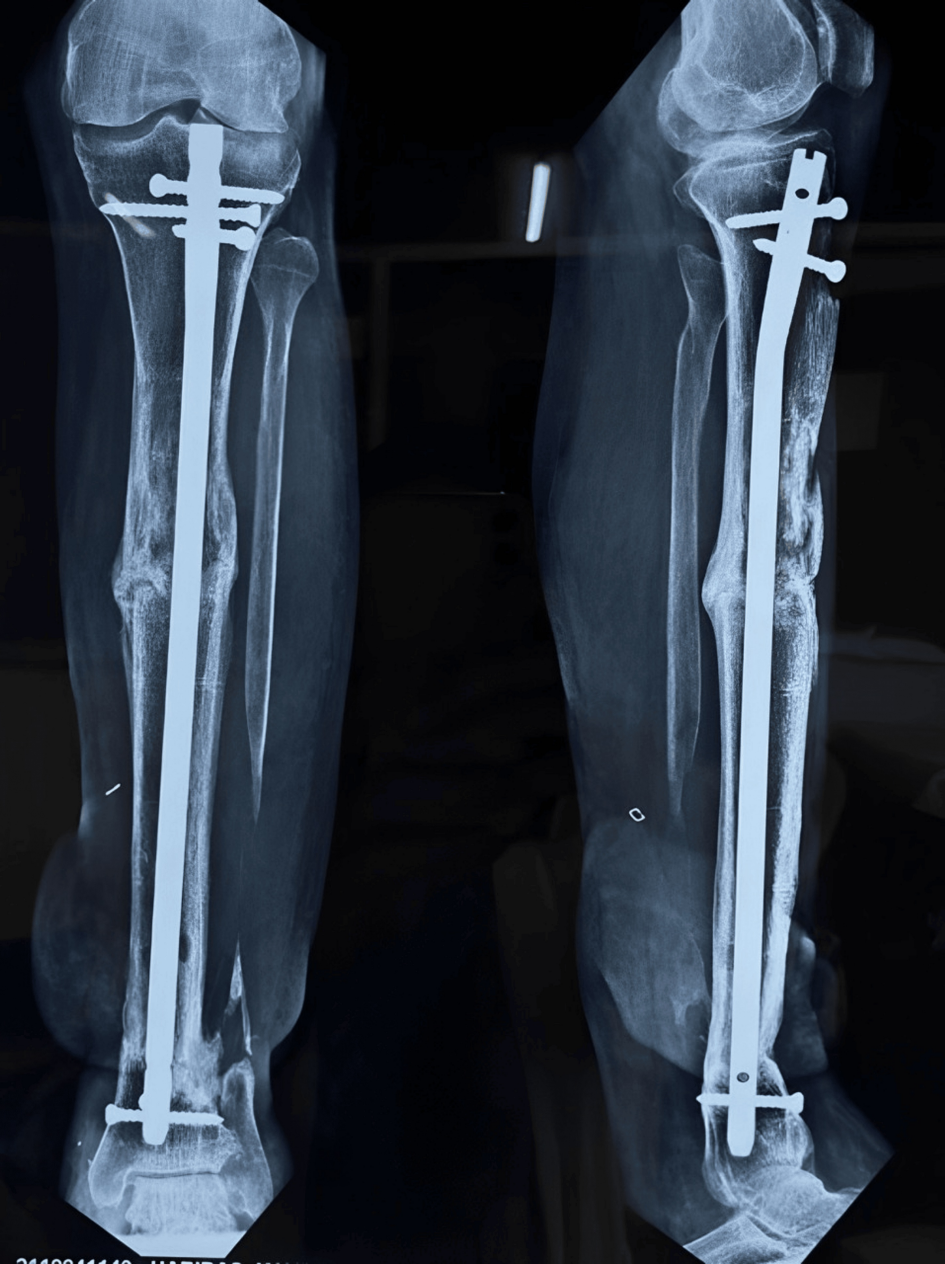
X-ray done at three months showing the initial signs of union
